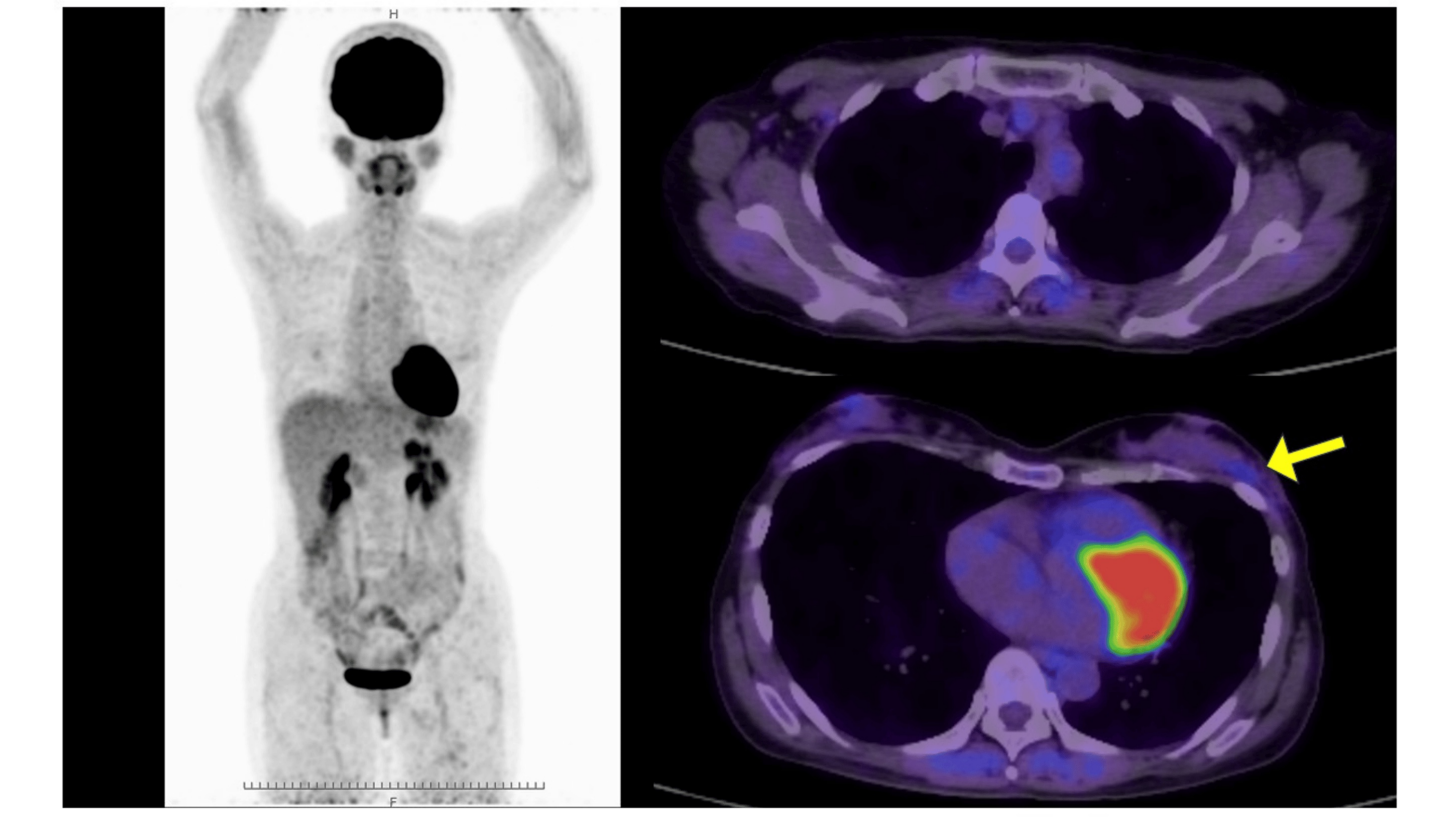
18F-FDG-PET/CT imaging after tumorectomy. ^18^F-FDG-PET/CT imaging after tumorectomy shows no abnormal 18F-FDG uptake throughout the body (a, b) except for slight accumulation (SUV max 1.6) in the left breast, which appears to be postoperative change (c, yellow arrow).
